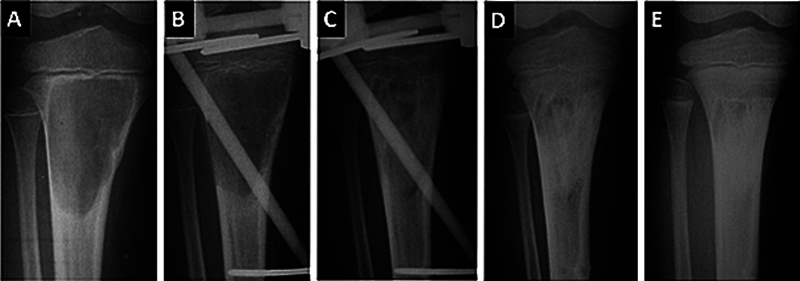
Serial radiographs of a 5-year-old patient with aneurysmal bone cyst in the tibia, adjacent to the growth cartilage (
